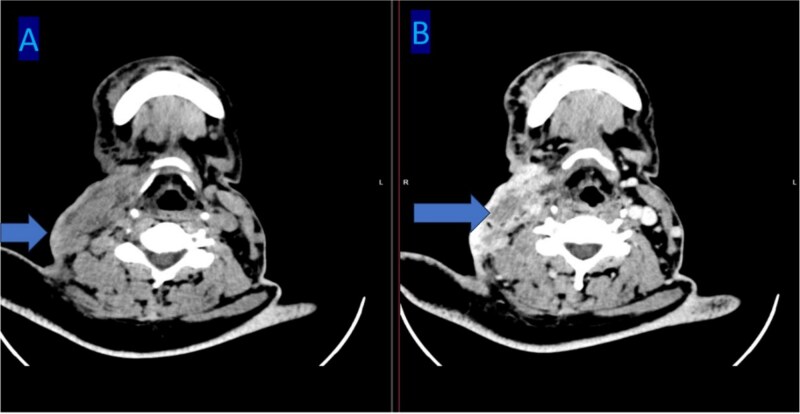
Multi slice head CT scan shows an enhancing soft tissue mass at the right parotid space, infiltrating the deep fat tissue extending between the inner border of the mandibular ramus and styloid process.
